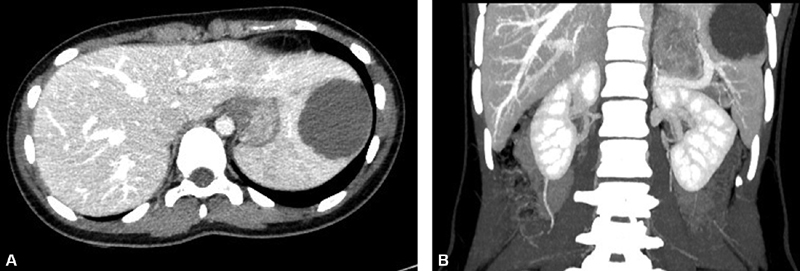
Computed tomography with intravenous contrast. Axial (
